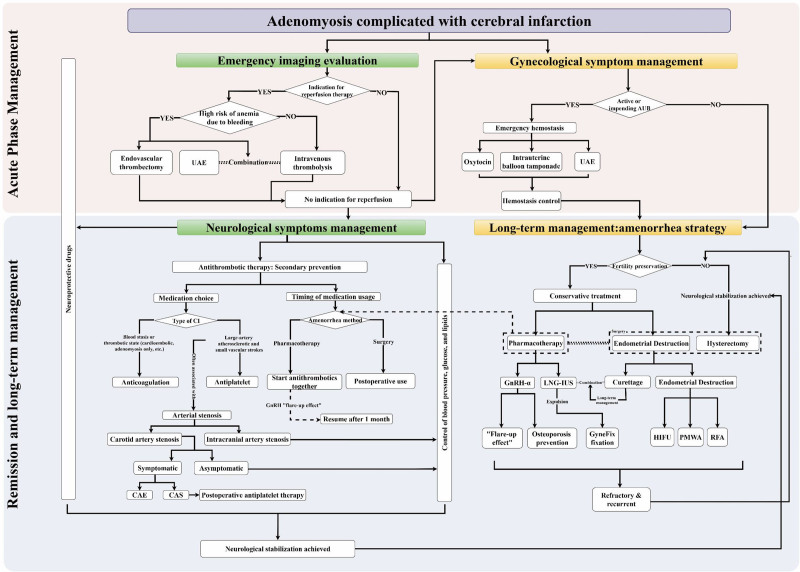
Multidisciplinary, staged management pathway for adenomyosis complicated by cerebral infarction. Acute-phase strategies focus on emergency imaging evaluation, reperfusion decision-making, hemostasis, and neuroprotection. Remission and long-term management emphasize amenorrhea induction (pharmacotherapy, LNG-IUS, endometrial destruction, or hysterectomy), individualized antithrombotic therapy, and control of vascular risk factors. The framework integrates gynecology, neurology, neurosurgery, and interventional radiology to balance hemostasis with thrombosis prevention. AUB = abnormal uterine bleeding, CAS = carotid artery stenting, CEA = carotid endarterectomy, CI = cerebral infarction, GnRH-α = gonadotropin-releasing hormone agonist, HIFU = high-intensity focused ultrasound, LNG-IUS = levonorgestrel-releasing intrauterine system, PMWA = percutaneous microwave ablation, RFA = radiofrequency ablation, UAE = uterine artery embolization.
